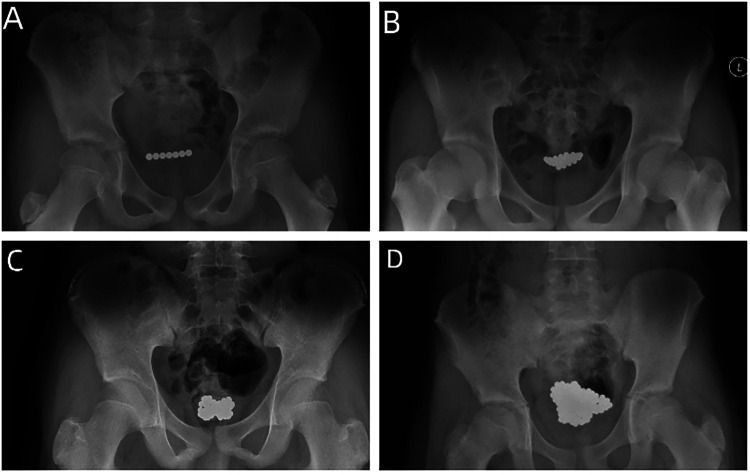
(A) A plain radiograph of the pelvis revealed a string of seven rounded high-density shadows in the pelvic region. (B) A plain radiograph of the pelvis revealed many rounded high-density shadows in a bead-like arrangement in the pelvic region. (C) A plain radiograph of the pelvis revealed many rounded high-density shadows in the pelvis above the pubic symphysis. (D) A plain radiograph of the pelvis revealed an irregular cluster of rounded high-density shadows in the pelvic region.
